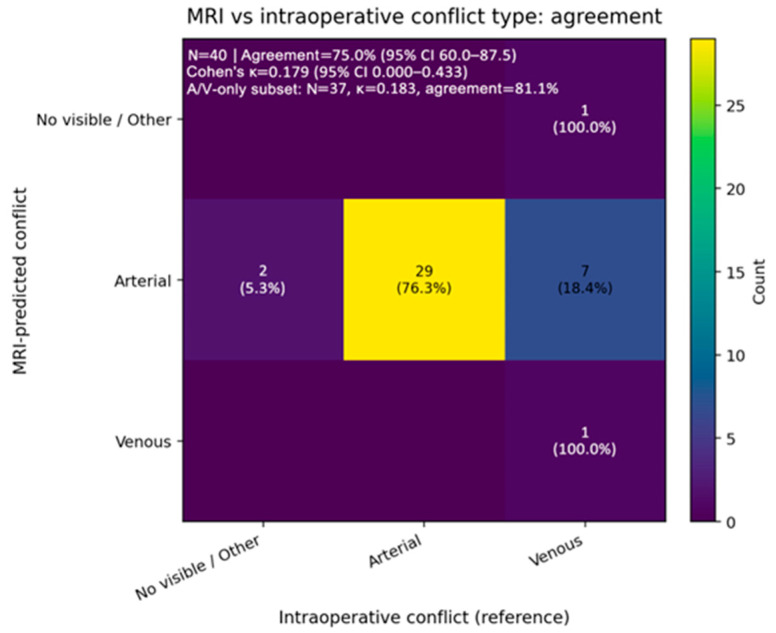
Preoperative MRI evaluation versus intraoperative conflict type in our study (95% CI 60.0–87.5).
